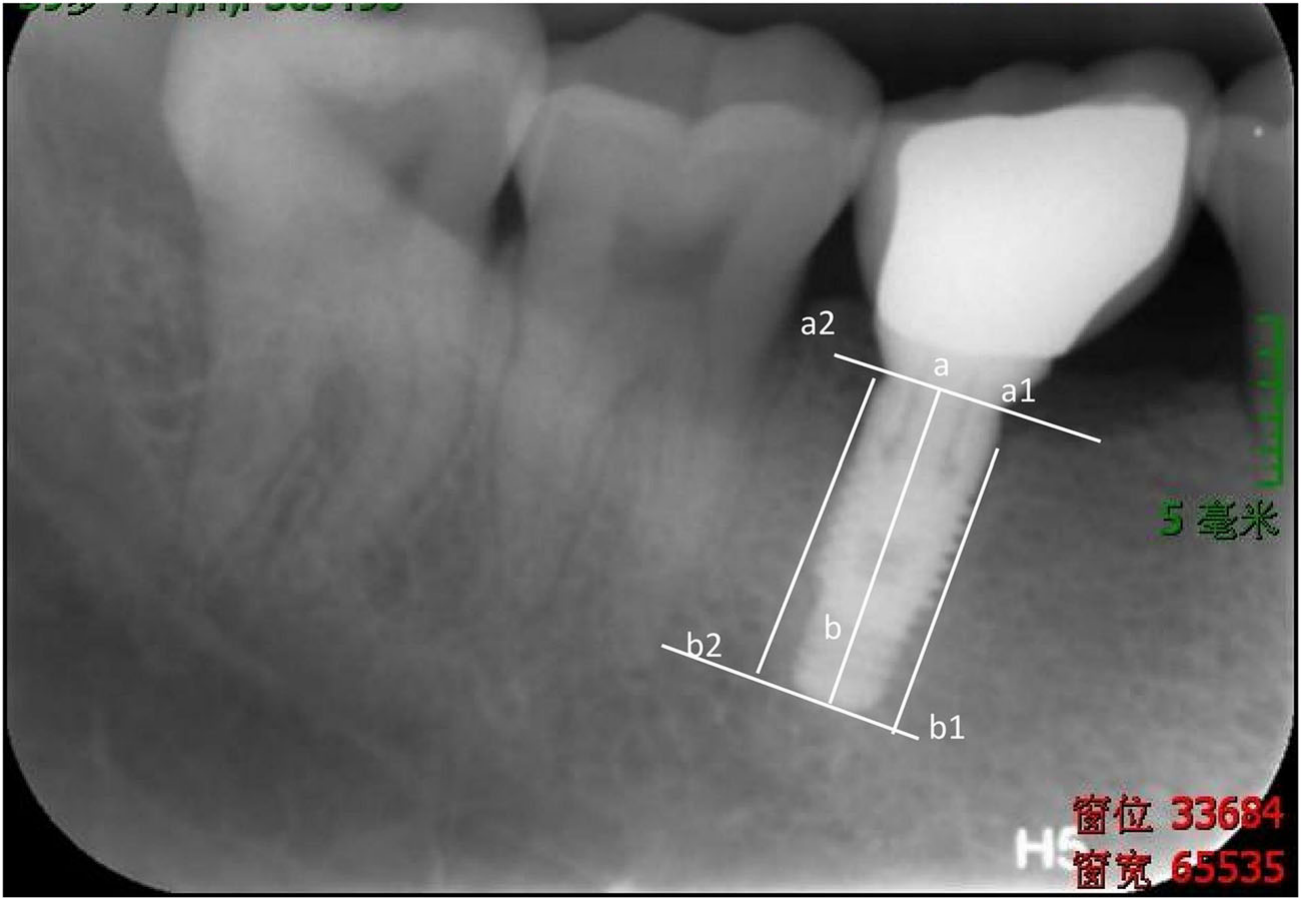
Radiographic measurements on MBL. Point b is the apical point of the end of the implant. Points b1 and b2 are the apical points on the mesial and distal sides of the end of the implant, respectively. Point a is the central point of the implant shoulder platform. Points a1 and a2 are the most apical initial points of the first visible bone contact on the mesial and distal sides of the implant, respectively. MBL at the mesial or distal side of the implant is calculated from the distance between a and b minus the distance between a1 and b1 or between a2 and b2, respectively.
